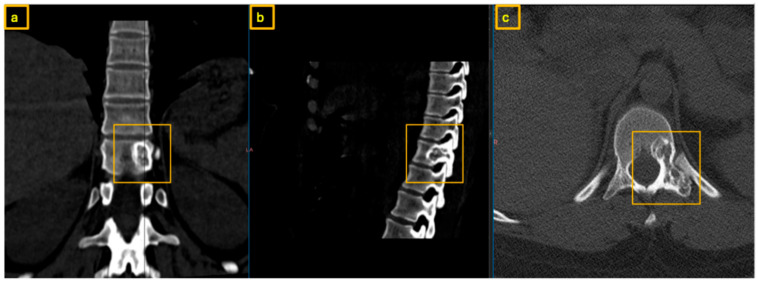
Preoperative CT of the thoracic spine. The yellow square shows the extent of the nidus within the posterior column, involving the root of the left T11 pedicle; (a) coronal view, (b) sagittal view, and (c) axial view.
